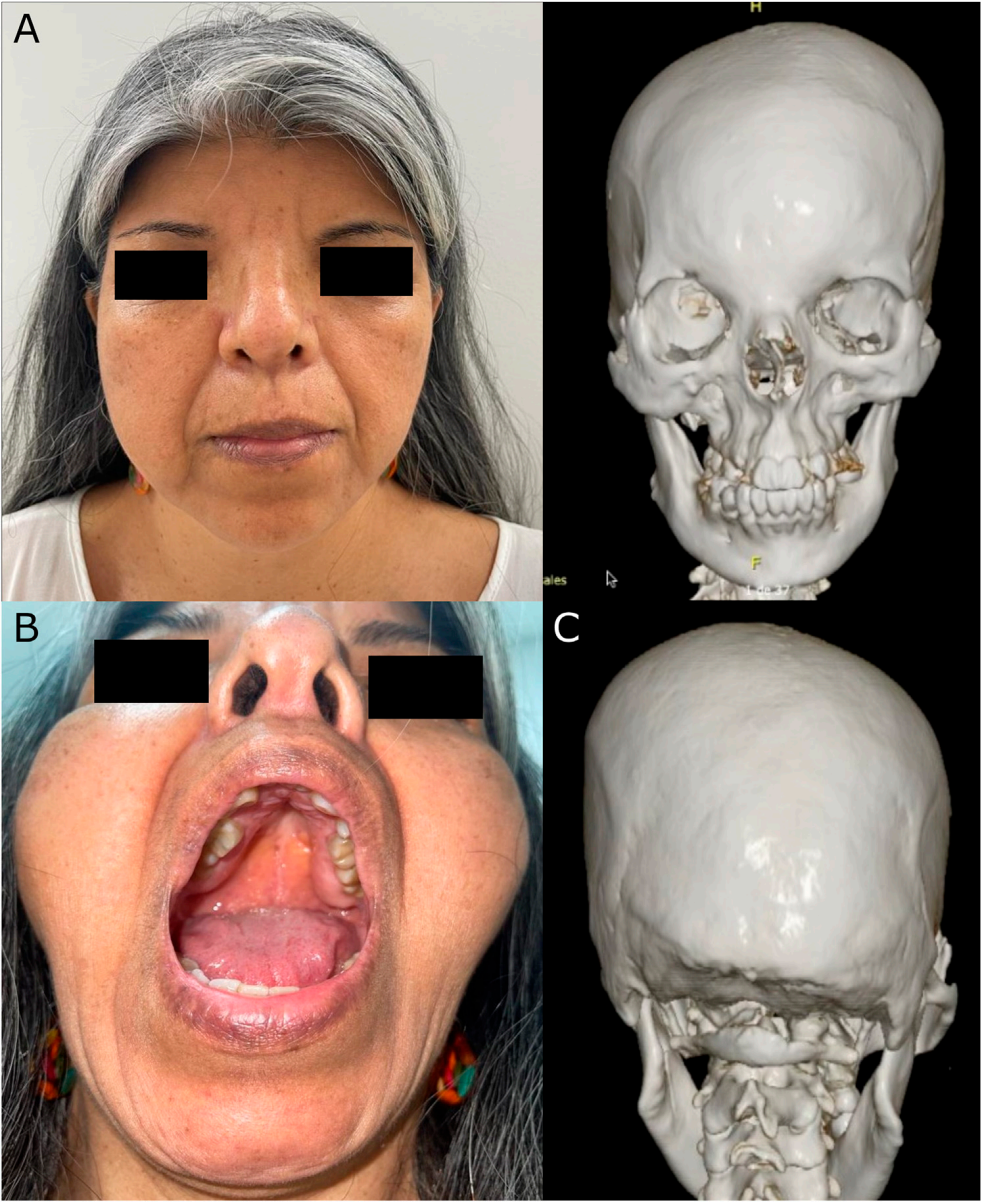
Cranial manifestations in the 54-year-old CFNS female patient. The figure shows (A) Facial dysmorphism (left) and frontal view of the 3D-CT showing facial asymmetry, dental crowding and the sinusoidal nasal septum with a large right bone spur (right); (B) high-arched palate, and (C) posterior view of the 3D-CT showing flat occiput and left parietal bone prominence.
